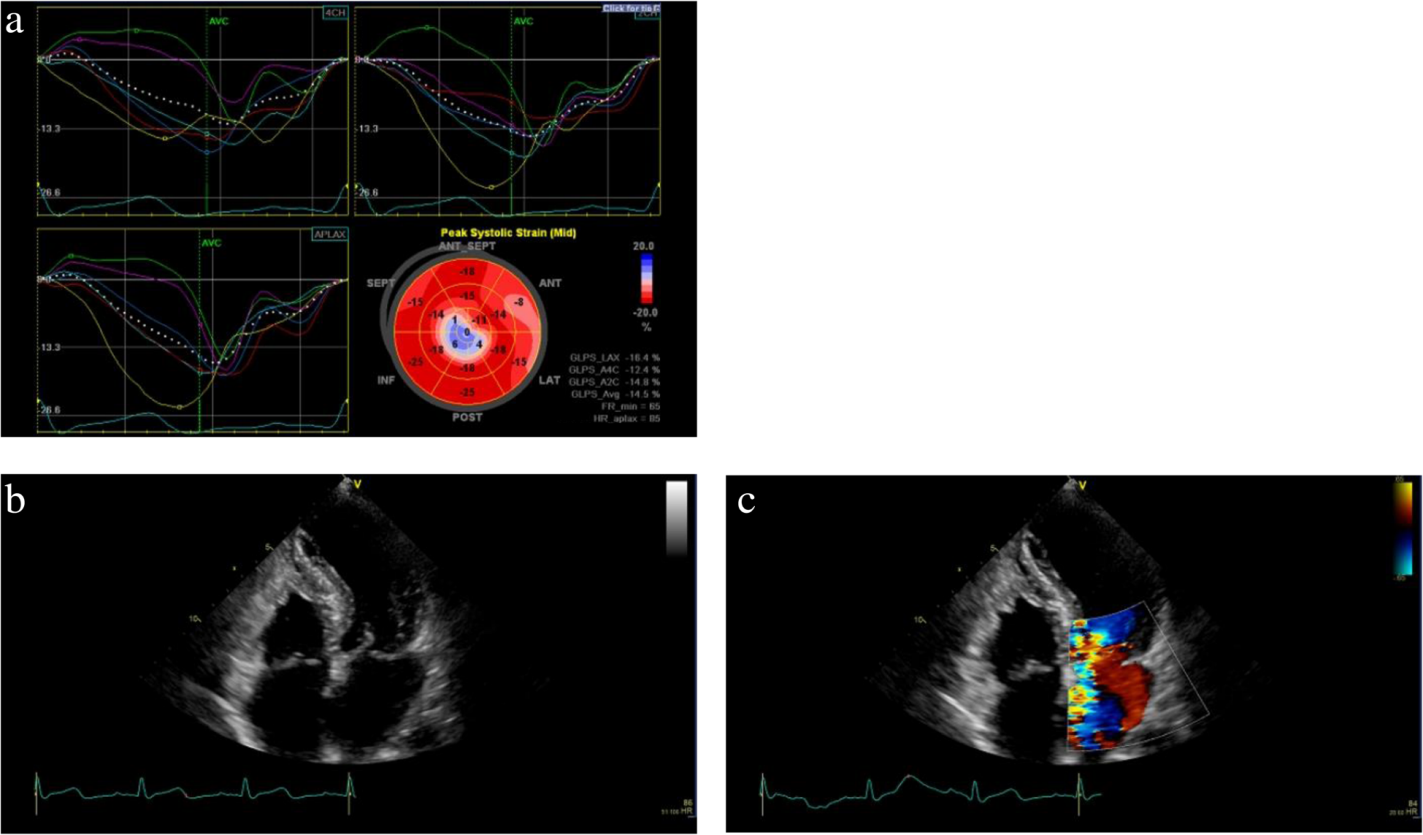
Transthoracic echocardiographic images obtained on admission showing (a) akinesis of the apical segments of the left ventricle, (b) tethering of the anterior mitral leaflet, and (c) eccentric severe mitral regurgitation
